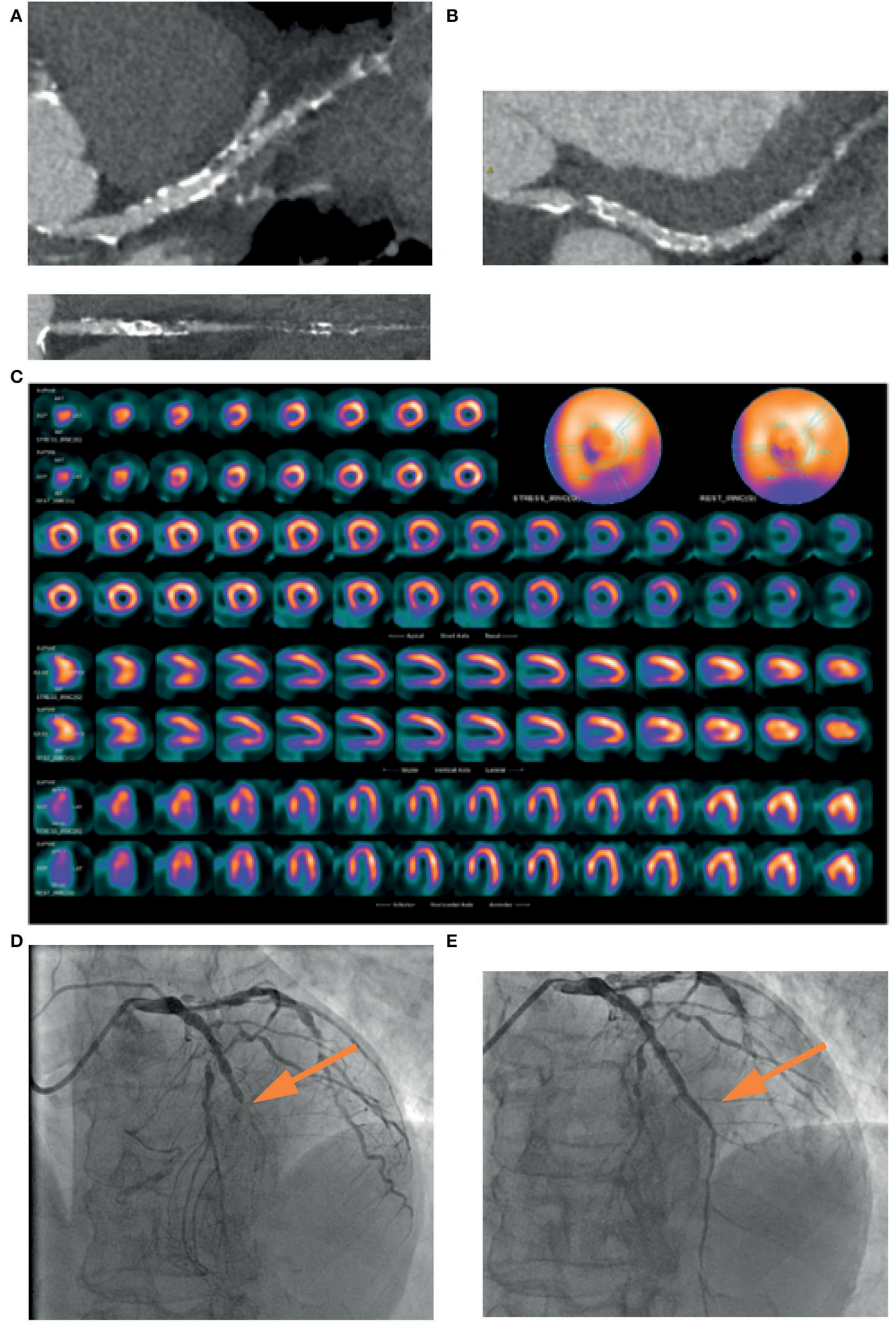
A 74-years-old patient, symptomatic for chest pain, performed a coronary CT, which showed a mild stenosis of left main and a diffuse severe stenosis of mid-distal left anterior descending (LAD) coronary artery (A). Circumflex artery had a significant ostial stenosis, with sub-occlusion of mid-distal portion (B) and right artery was occluded at the mid portion (C). SPECT showed perfusion defect at the apical septum and at infero-lateral mid-basal wall (D). Coronary angiography confirmed the presence of sub-occlusion (*yellow arrow*) of the middle section of the LAD coronary artery (E), treated with coronary angioplasty and placement of a medicated stent (*yellow arrow*), with a good final angiographic result (F).
